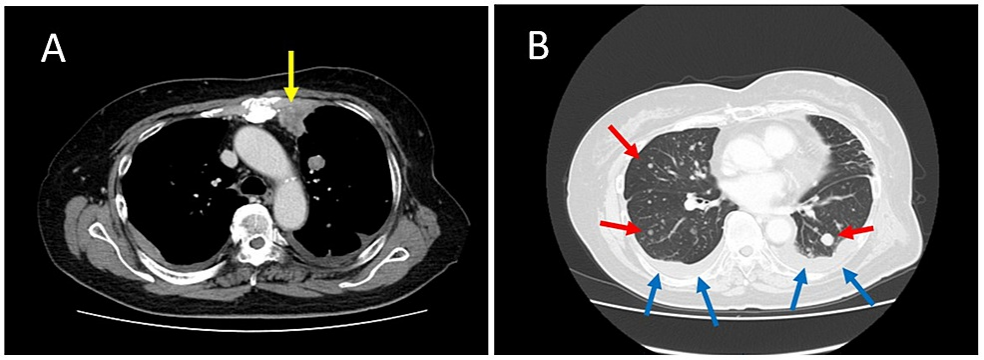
CT scan images. (A) The yellow arrow indicates the internal thoracic lymph node metastasis. (B) The red arrows indicate multiple lung metastases, and the blue arrows indicate pleural effusion. CT: computed tomography
